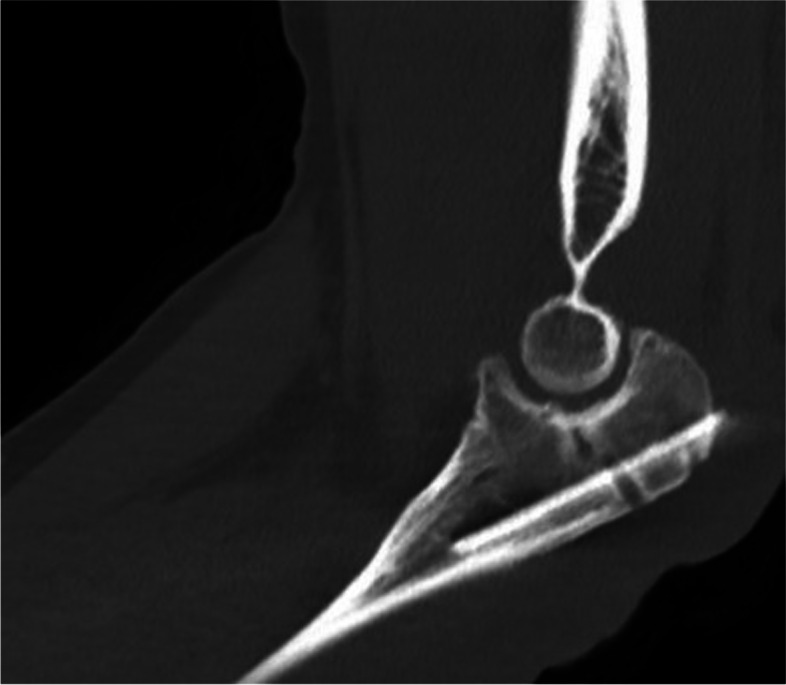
Postoperative CT-scan showing the centered joint, the remaining screw and screw holes after hardware removal
